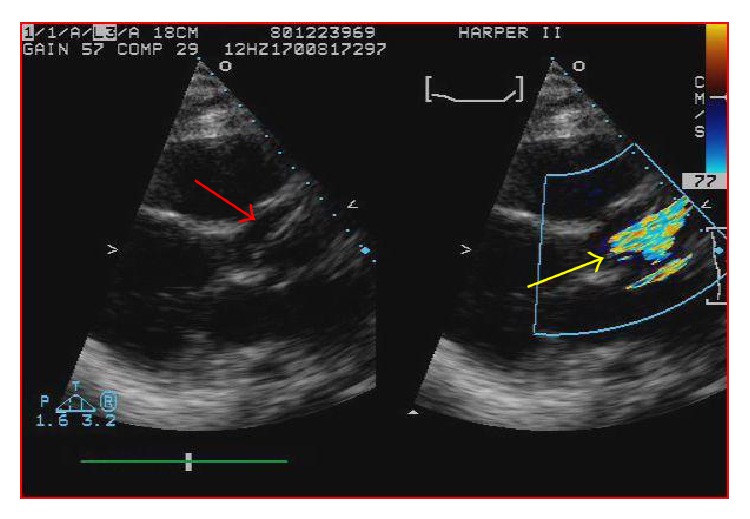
After echocardiographic guidance the Impella inlet is properly positioned above the aortic valve (red arrow). Supravalvular turbulence is indicated by the yellow arrow.
